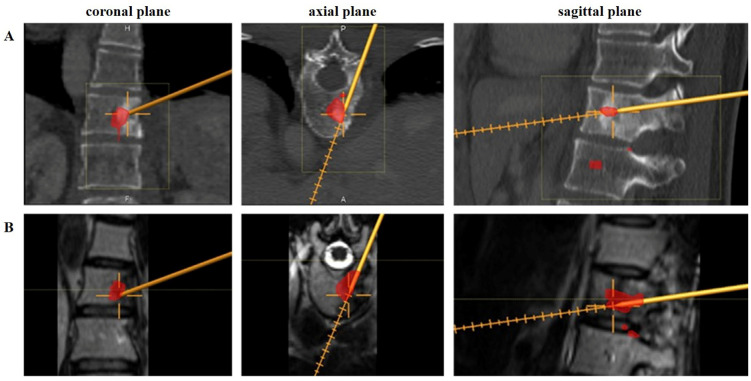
A 54-year-old patient with a recurrence of chondrosarcoma at the level of the thoracic spine (Th 12). The area corresponding to the FDG-avid lesion in the thoracic spine (Th 12) was marked with Smartbrush software for navigated biopsy, as visualized in (A) CT and (B) MR imaging.
